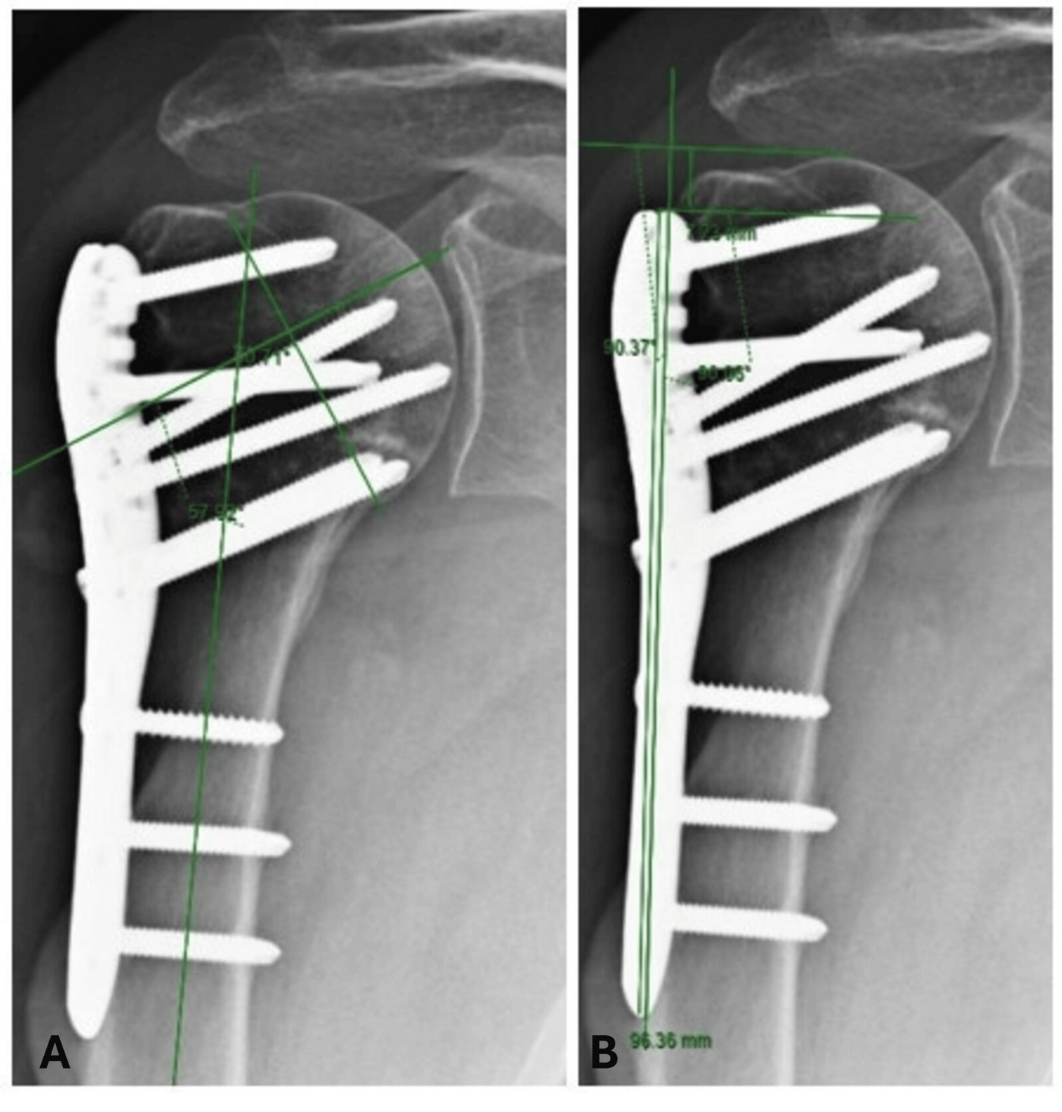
Postoperative Radiographs: Radiographic image of the postoperative follow-up after calculation of the head-shaft angle (HAS) (A) and calculation of humeral height (HH) (B).
